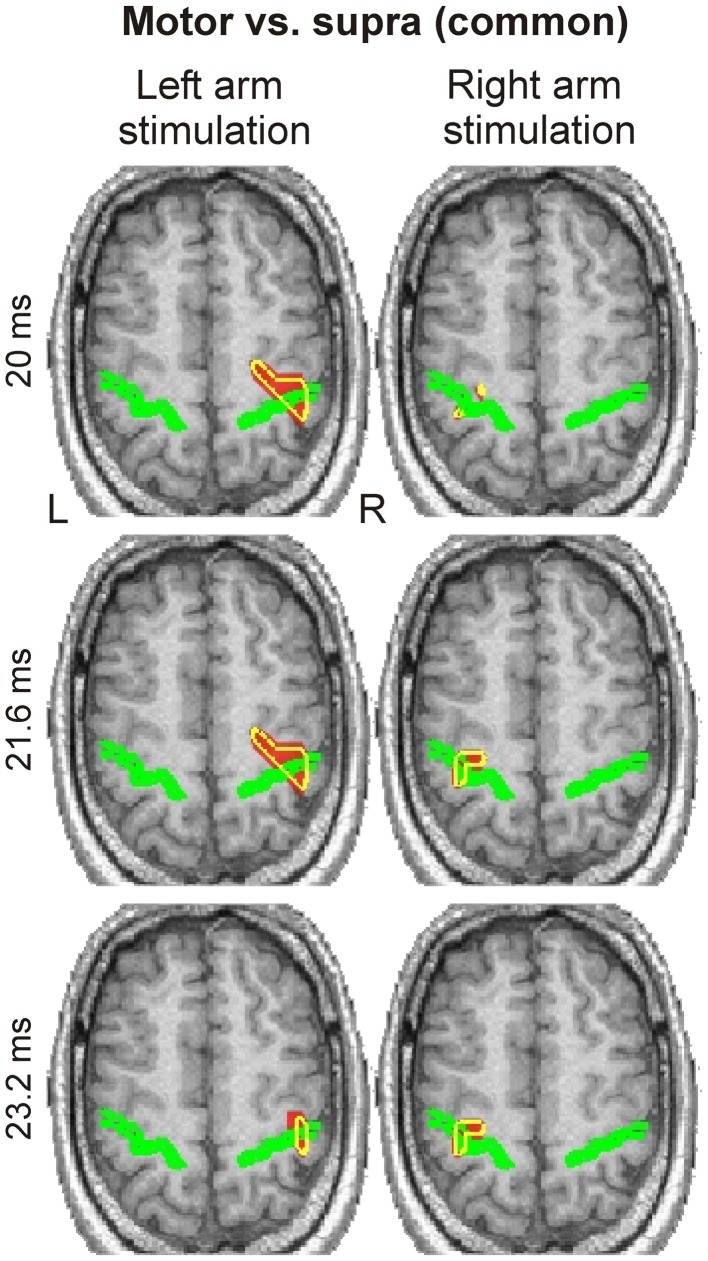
Spatio-temporal grand activation maps derived from “Motor vs. Supra” contrast are shown for left (left column) and right (right column) arm stimulation at three latencies (indicated at the left side of each row). The common across all three subjects activations are projected on the MRI of the blind subject. The axial slice of MRI best covering the central sulcus, indicated by a green outline, is shown. Yellow contours encompass regions with statistically significant (*P* < 0.0001) increases in activity in all three subjects.
